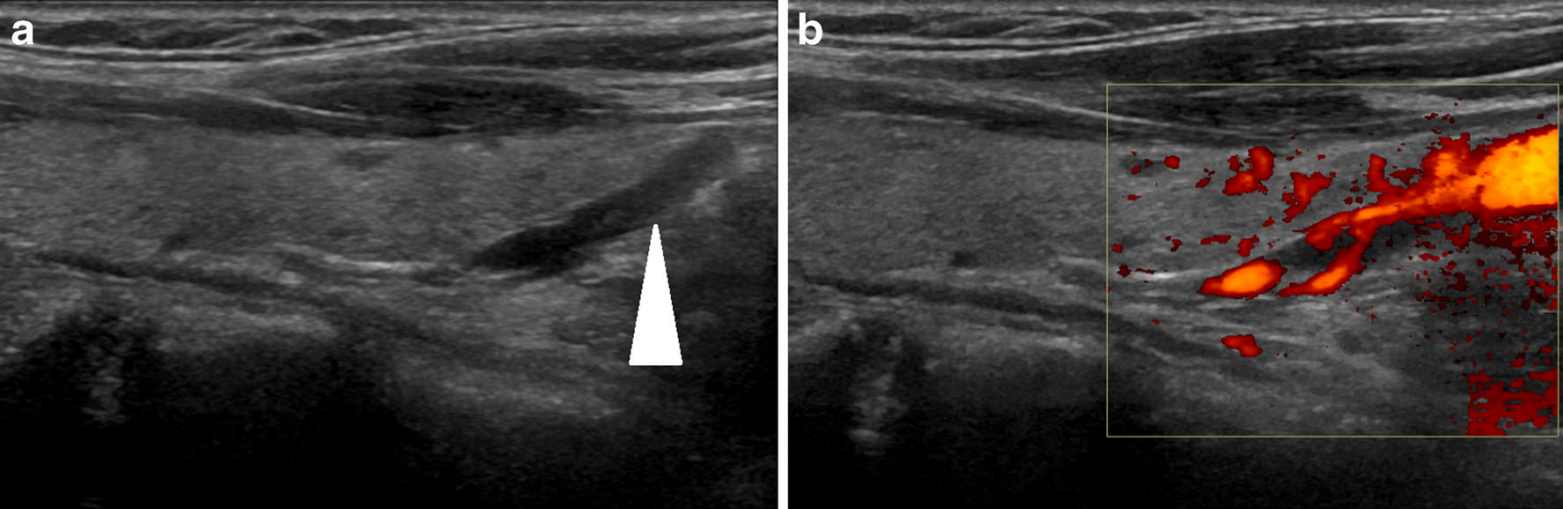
a) Longitudinal ultrasound image of a parathyroid adenoma in a 54-yr-old female with HPT-JT. The adenoma (arrowhead) corresponds to the elongated hypoechoic structure caudal to the thyroid. On application of colour Doppler (b) the lesion is demonstrated to be hypervascular
